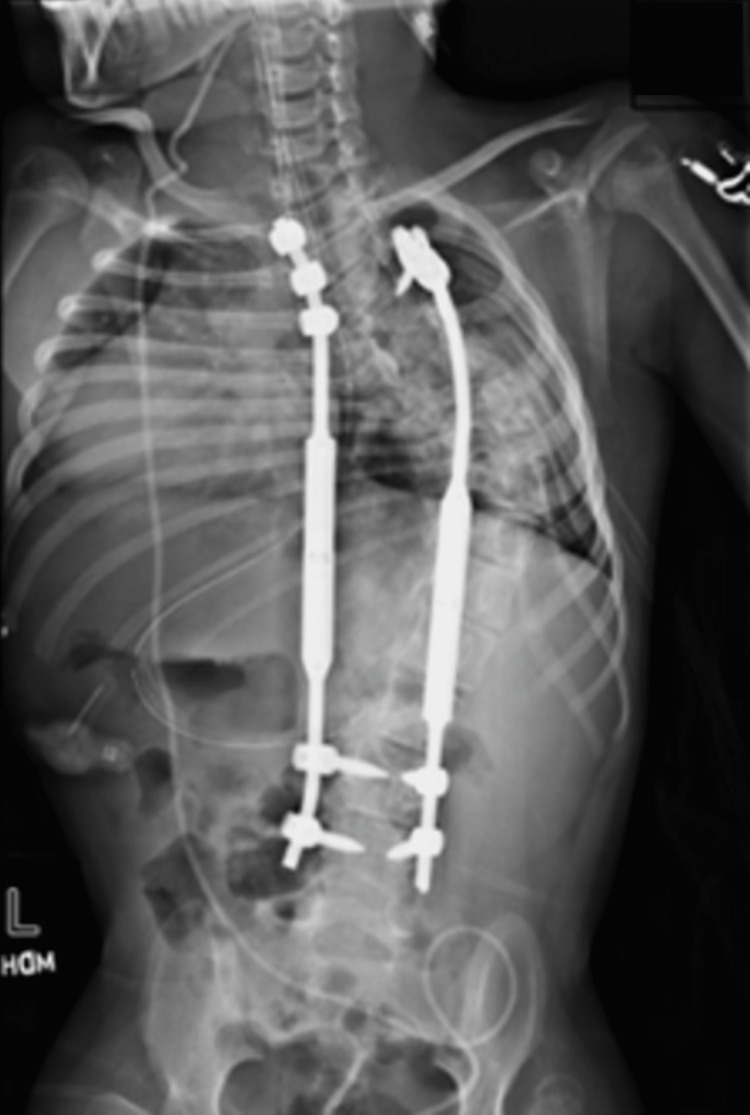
Posterior-anterior plain radiograph performed after index surgery for MAGEC rod placements at eight years of age.
